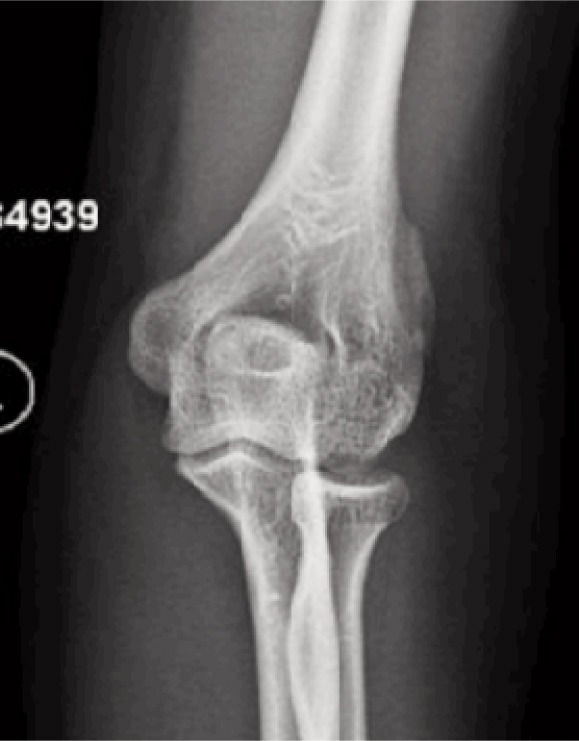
Follow up radiograph at 14 weeks shows good callus and bony union.
